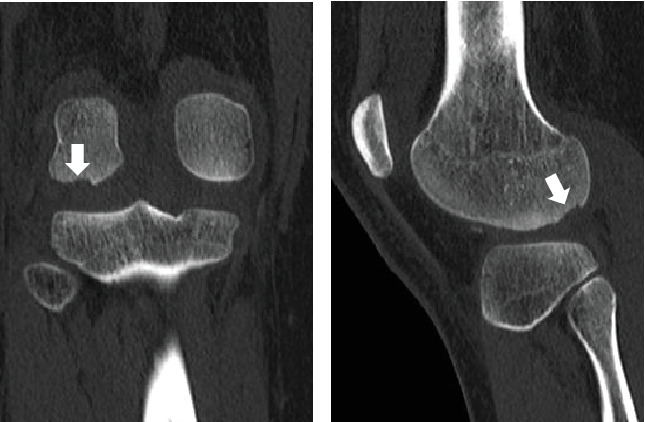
CT images of an osteochondral defect in the posterior portion of the lateral femoral condyle (LFC), as indicated with the arrow. (a) Coronal section and (b) sagittal section.
